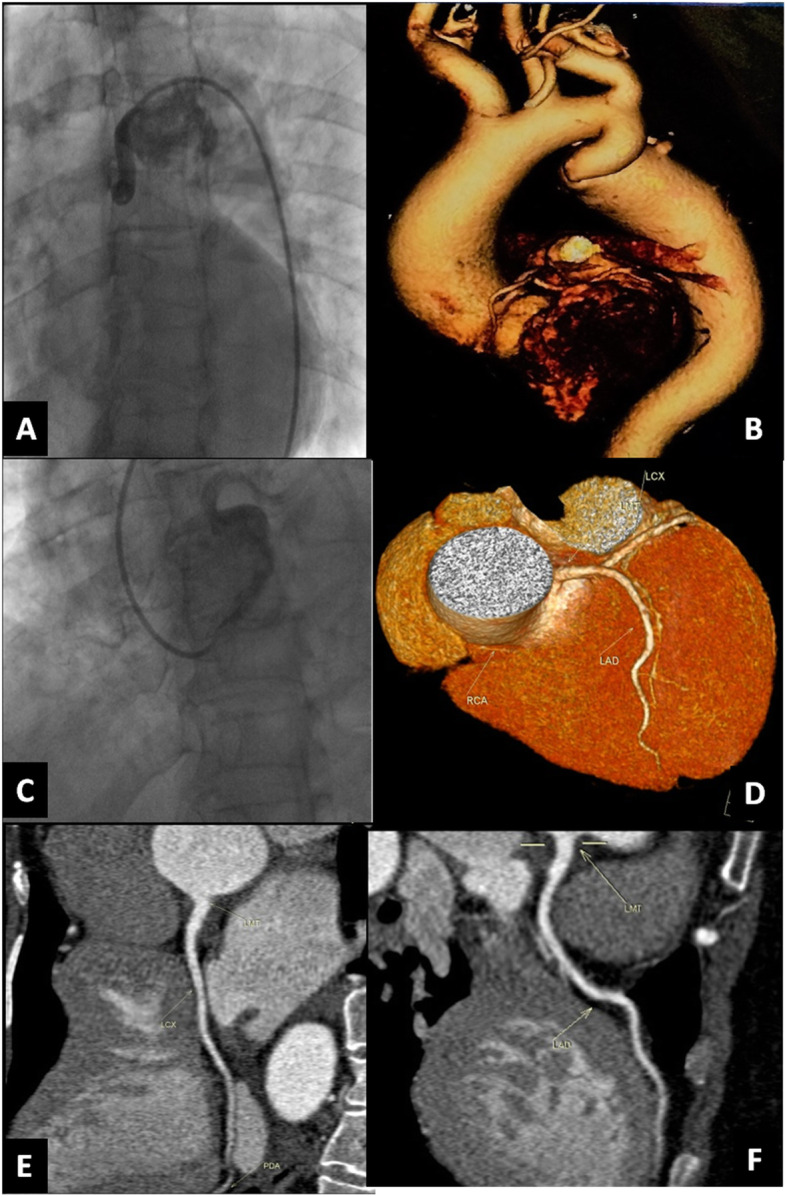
Demonstration of a case of aortic coarctation with the inability to pass the catheter. A Difficult passage of catheter due to aortic coarctation. B VRT of CT aortography reveals aortic coarctation just distal to the origin of the left subclavian artery. C Non-selective injection of contrast by catheter due to the difficult engagement of coronaries. D VRT of CCTA reveals the anatomy of different coronary arteries. E, F MPR of CCTA reveals normal LCX and LAD, respectively
